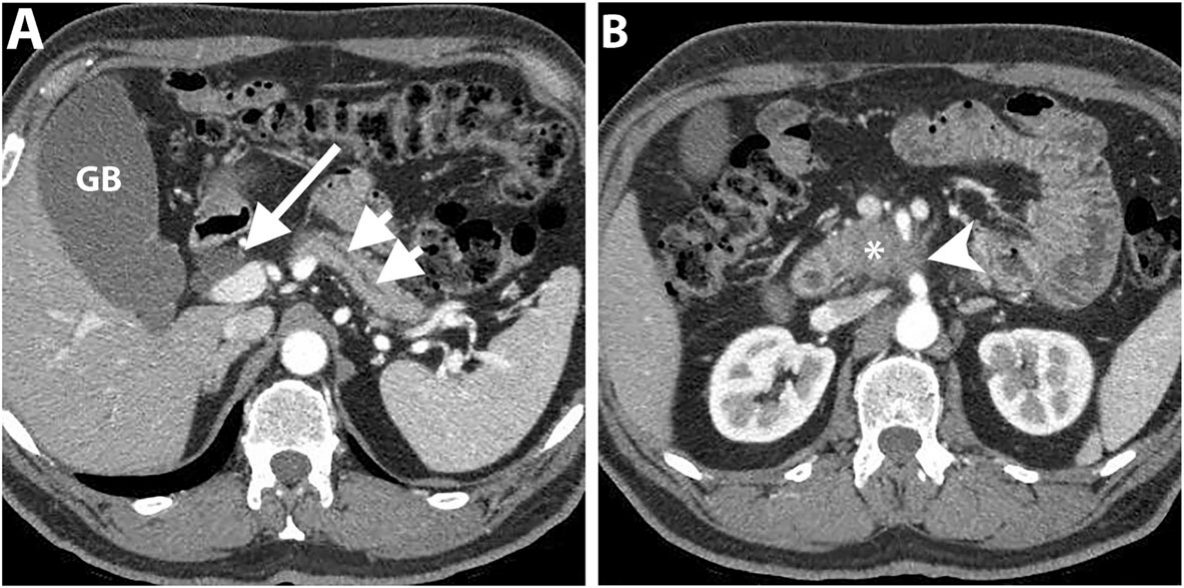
A 63-year-old man with locally advanced PDAC in uncinate process. Axial pancreatic phase CT images (a and b) show a mass (arrowhead) in the uncinate process encasing SMA with mild upstream dilatation of CBD (arrow) and MPD (short arrows) and associated with mildly distended gallbladder (GB)
